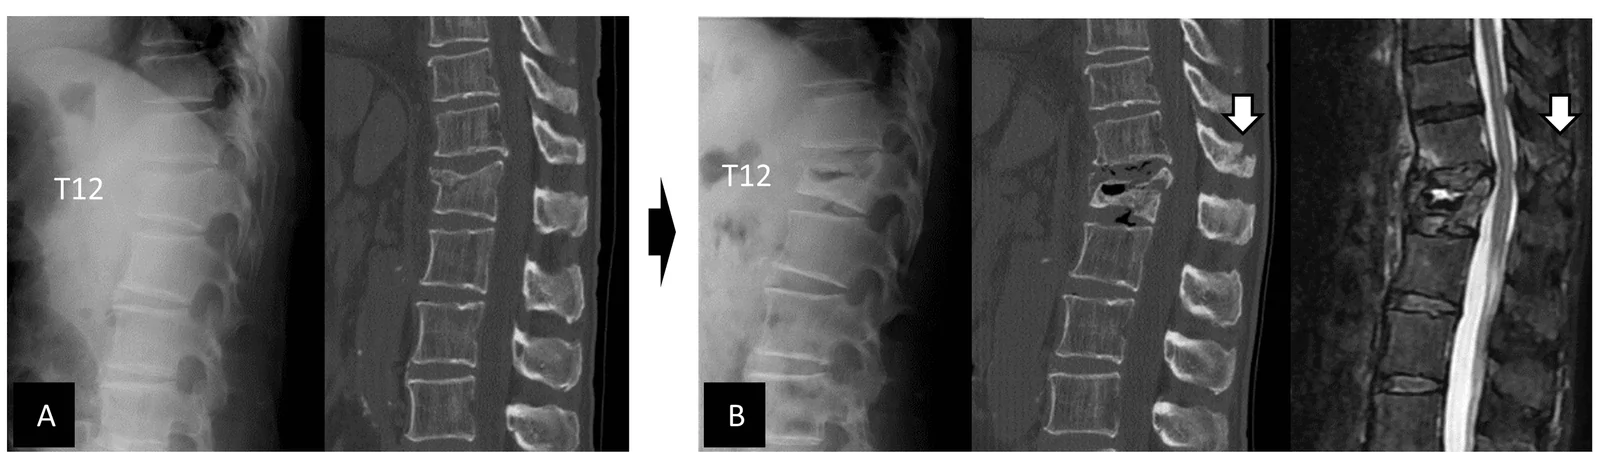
Serial images demonstrating delayed development of a spinous process fracture during progression of vertebral collapse A) Radiograph and computed tomography (CT) at two days post-injury. A fracture of the T12 vertebral body is observed with no injury to the posterior structures; B) Radiograph, CT, and magnetic resonance imaging (MRI) at five months post-injury. A cleft formation is noted in the T12 vertebral body. A vertical fracture is observed in the spinous process of T11 (white arrow).
